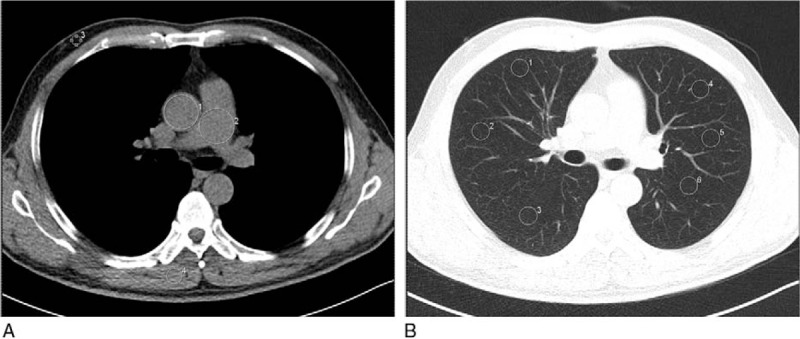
An example of ROI of measurements on transverse unenhanced multidetector CT images. For all measurements, the size, shape, and position of the ROIs were kept approximately constant across patients. (A) ROIs manually drawn on the descending aorta (ROI 1), pulmonary trunk (ROI 2), subcutaneous fat of the anterior abdominal wall (ROI 3), paraspinal muscle (ROI 4) and air (ROI 5); (B) ROIs manually drawn on the pulmonary parenchyma (ROIs 1–6). CT = computed tomography, ROI = range of interest.
